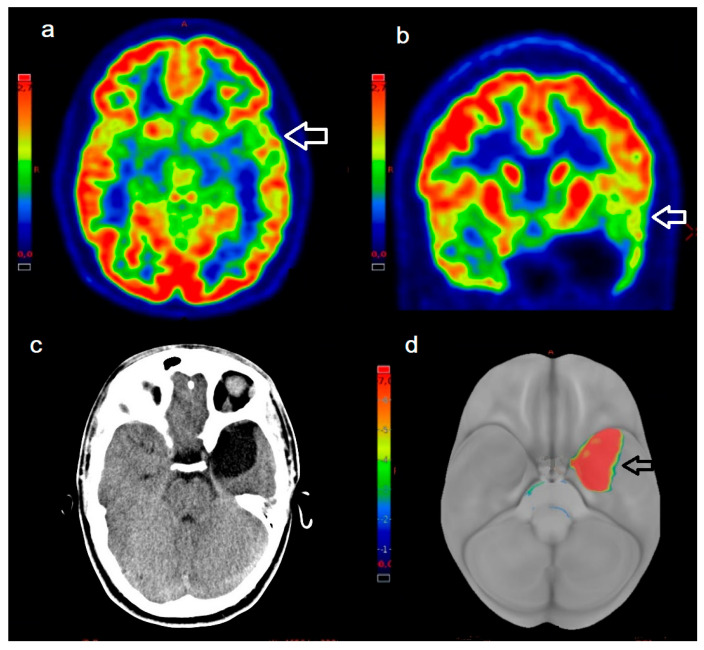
A 13-year-old male patient with a left temporal lobe cyst and epilepsy characterized by absence seizures and clonic–tonic manifestations, submitted to ^18^F-FDG aPET. Axial (a) and coronal (b) PET images show asymmetric tracer distribution in the left temporal region near the supero-lateral margin of the temporal cyst (white arrows), clearly visualized on the co-registered CT scan (c). Qualitative evaluation using the Paldino score yielded a value of 2 (i.e., possible laterality with unclear borders), with full inter-observer agreement. Panel (d) shows a 3D volume rendering of the Z-score in the left temporal lobe (−0.6; black arrow). The patient underwent surgical removal of the cyst, which was causing an irritative effect on the adjacent parenchyma, resulting in symptomatic improvement.
